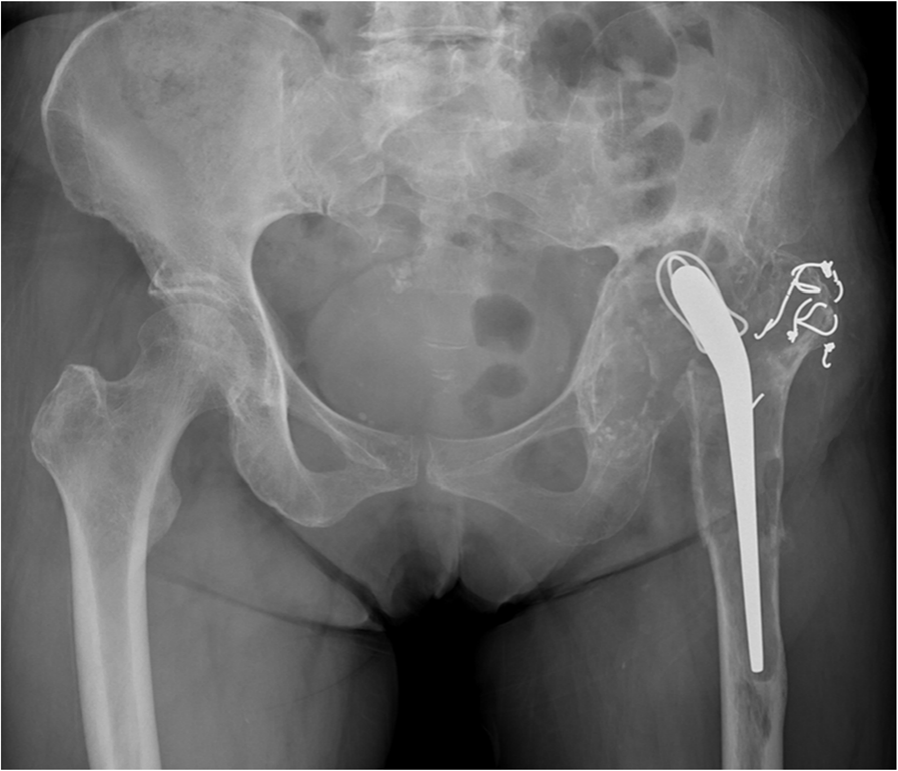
A plain anteroposterior radiograph of the bilateral hip at the patient’s first visit showing extensive osteolysis around the implant and upper migration of the left femur
